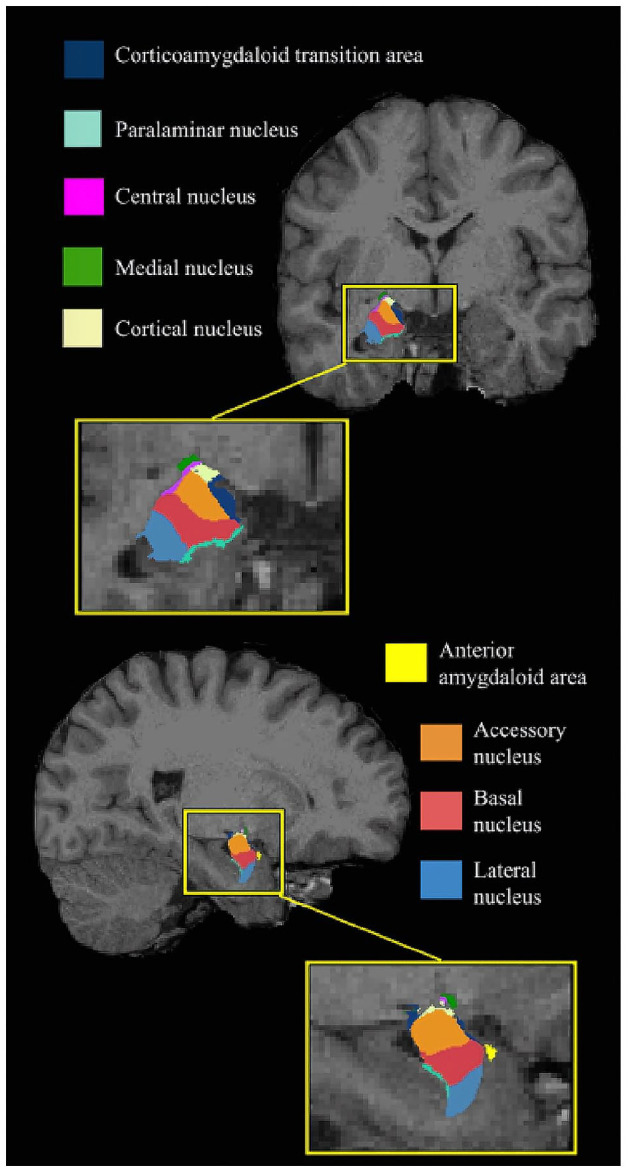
Amygdala atlas registered to a T1-weighted image obtained in a
